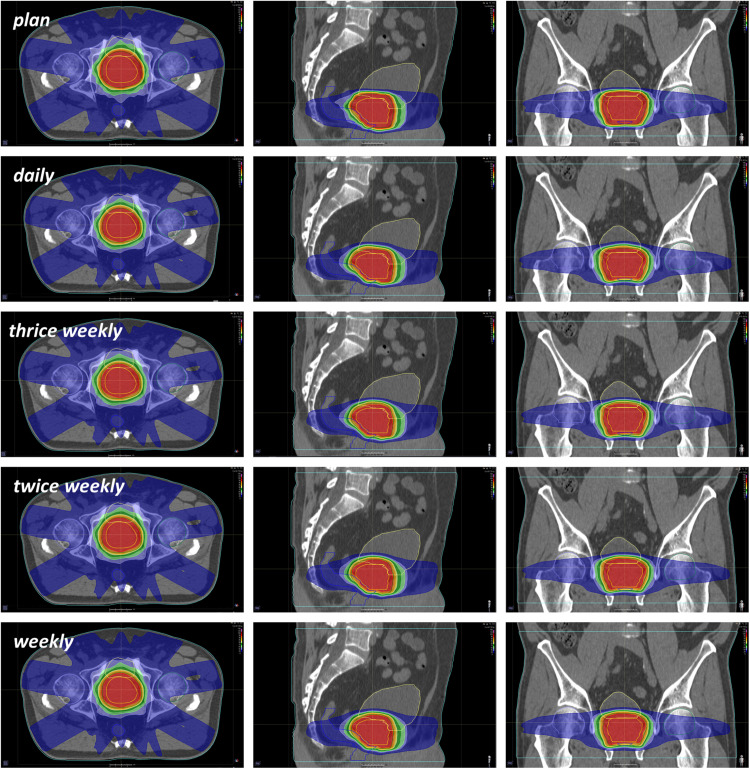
Representative CT images demonstrating the distribution of planned and applied doses in relation to the positional imaging frequency.
